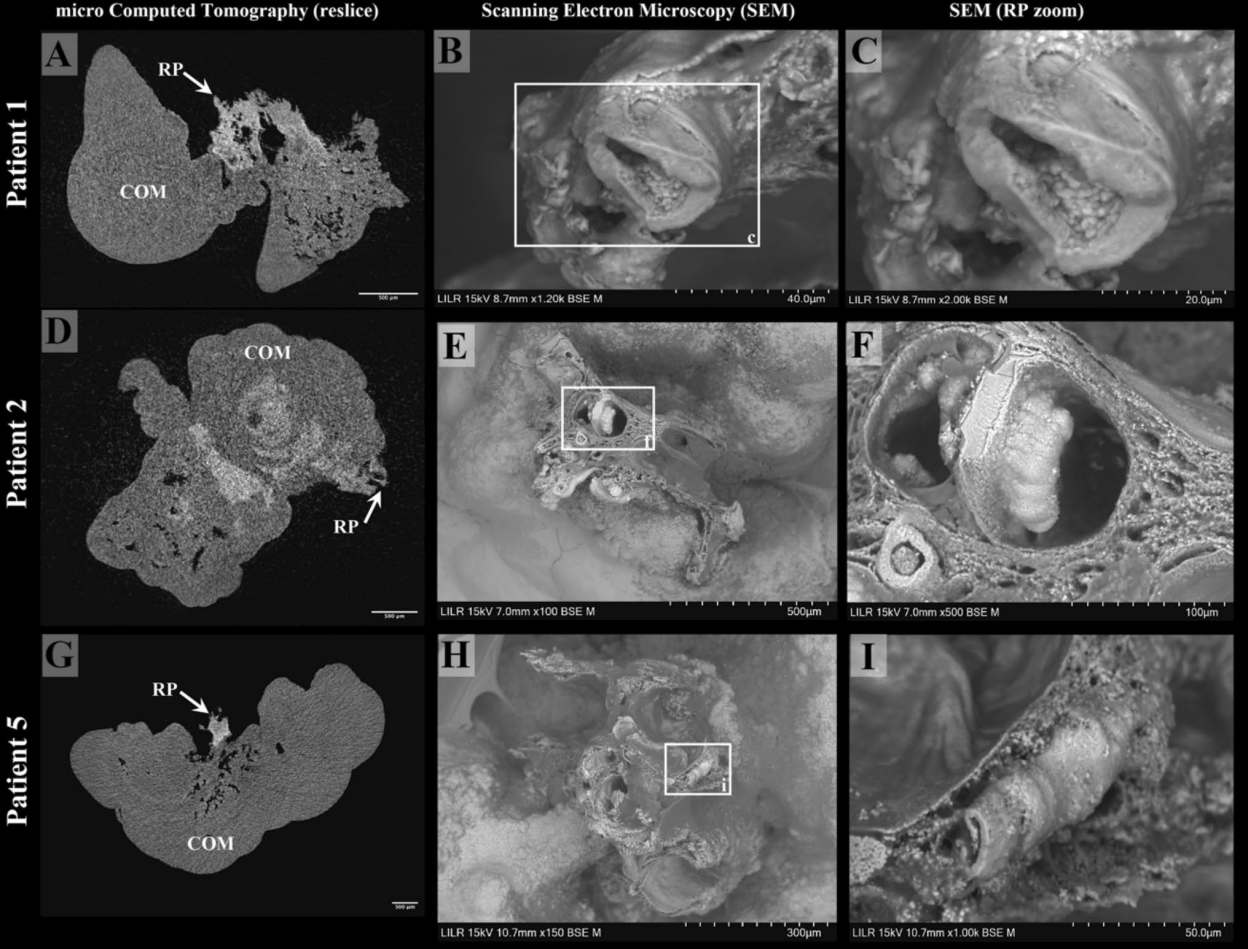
Micro CT and SEM analysis of Randall’s plaque (RP) kidney stones from patients 1, 2, and 5. Micro CT reslice images showing calcium phosphate (CaP, apatite) in RP regions (A, D, G). SEM images reveal mineralized tubules, potentially originating from thin loops, collecting ducts, or ducts of Bellini (B, E, H). Tubules are often covered with collagen fibrils, with dense or particulate mineral deposits (C and I). Example of an elliptical mineralized tubule (~ 35.18 µm × 21.7 µm), likely from the loop of Henle (C). In Fig. 1F, top views of RP areas in patient 2 show ruptured and dilated tubules (~ 30–35 µm diameter), with some larger dilated tubules (~ 90–110 µm). Patient 5’s RP plaque features broken tubules (26–40 µm) and large papillary duct casts (~ 100–113 µm) (H and I)
